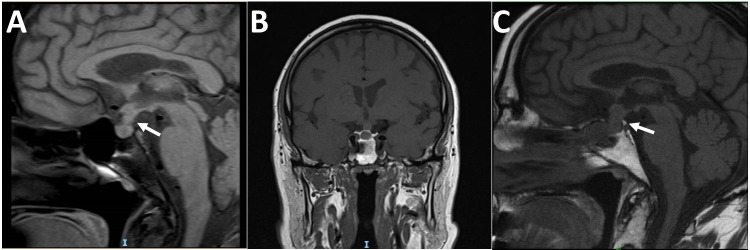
Post-contrast T1-weighted pituitary magnetic resonance (MR) images in one patient with IgG4-RH. A Pre-treatment sagittal section scan showing a pituitary adenoma 15 × 7 × 8 mm (height × width × length) with sellar extension (white arrow). Pituitary stalk is thick and bulges into infundibular recess, posterior bright spot of neurohypophysis is lost. B Post-treatment coronal section scan at 5 months after treatment with oral glucocorticoids over 4 weeks did not show any reduction in mass and visual loss persisted. C Post-operative sagittal section scan at 1 year following pituitary biopsy shows reduction in pituitary mass and residual thick pituitary stalk (white arrow)
